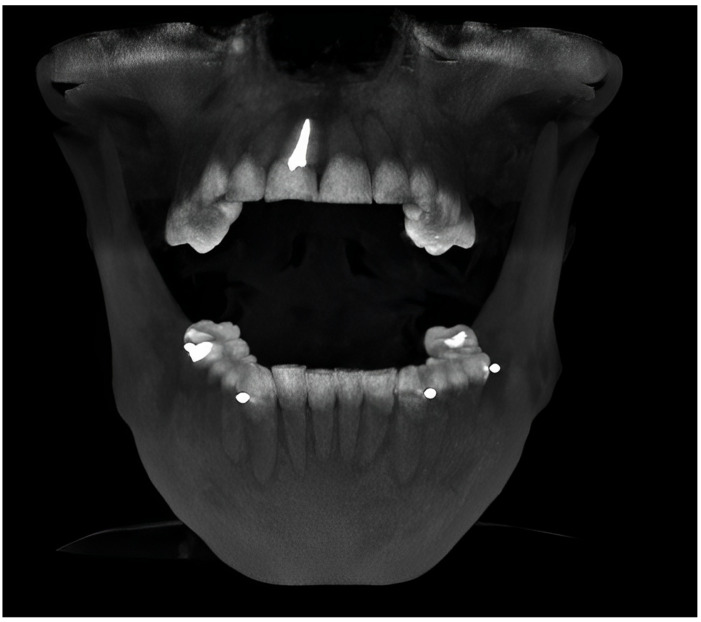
The pre-CBCT was executed in maximum opening position.
